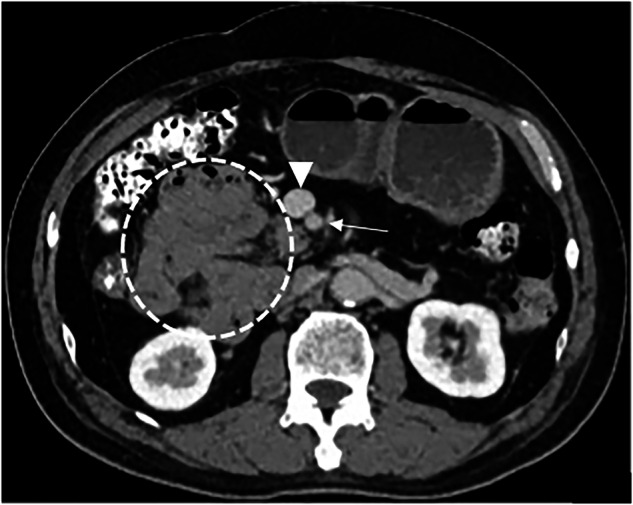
Fifty-nine-year-old man with midgut malrotation. Axial contrast CT showed the jejunum situated in the right abdomen (circle); the SMV (solid triangle) positioned anterior to the SMA (thin arrow)
